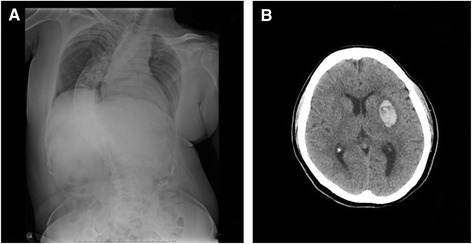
Initial computed tomography (CT) and spinal radiography performed in a 55-year-old woman presenting with acute left hemiparesis. A) Posterior-anterior spine radiography demonstrating scoliosis. B) Initial CT demonstrating putaminal hemorrhage.
